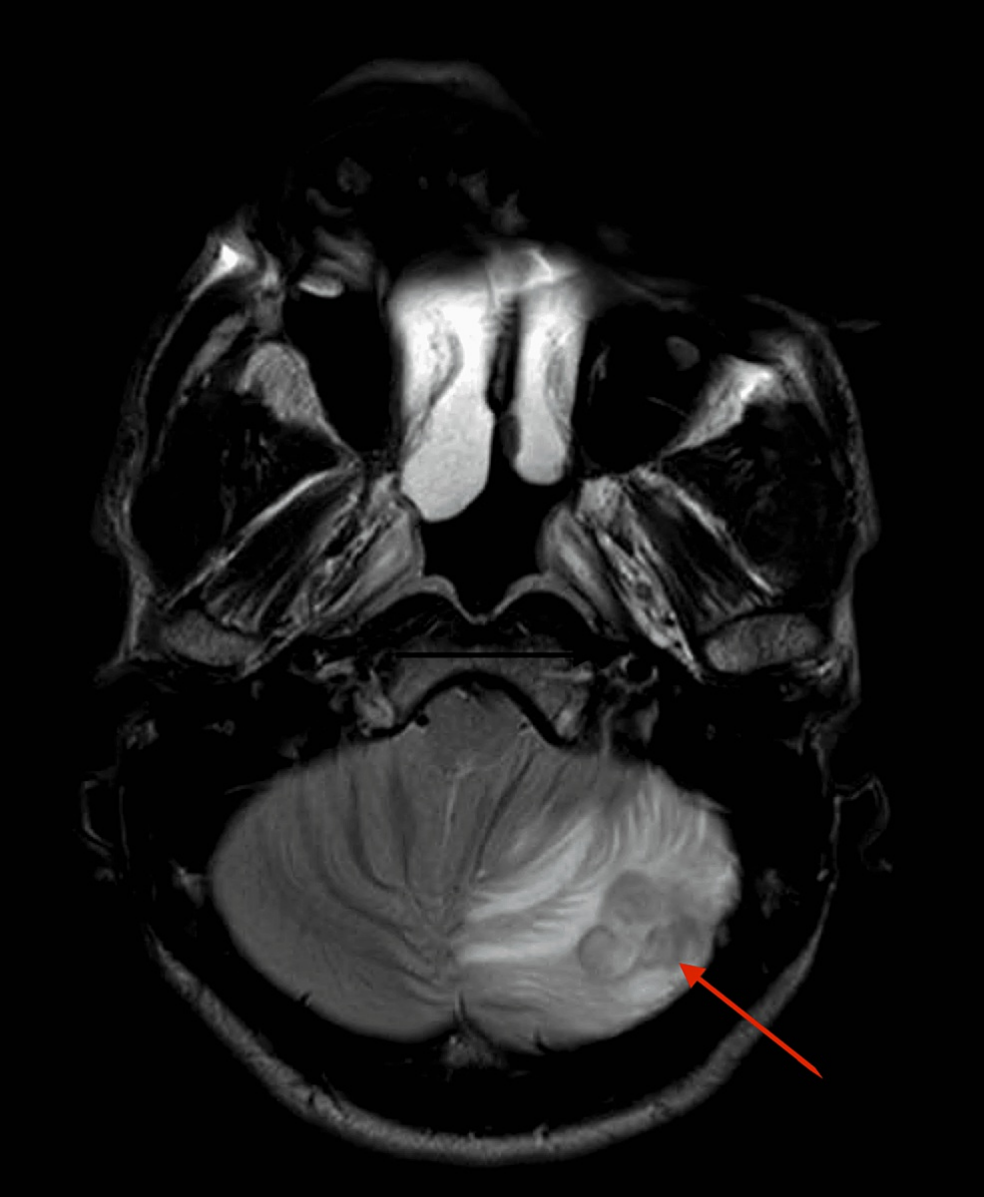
T2 series of magnetic resonance imaging (MRI)
